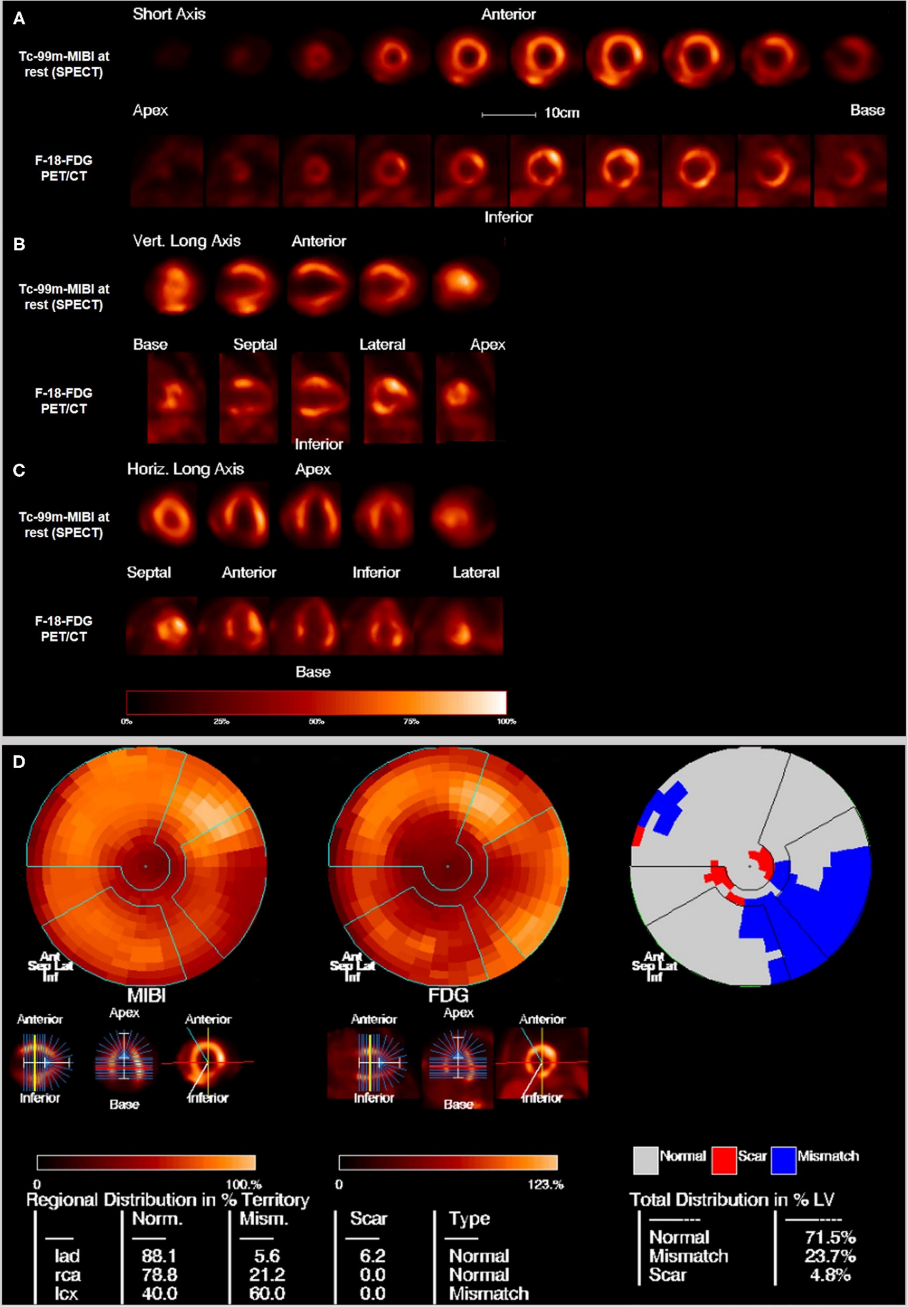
Example of hibernating myocardium: 76 years old male with a perfusion defect on the posterolateral wall in the Tc-99m-MIBI rest scan that shows FDG uptake in the viability F-18-FDG-PET/CT scan resulting in the diagnosis of hibernating myocardium. (A) Short axis, (B) vertical long axis, and (C) horizontal long axis. The upper rows show the perfusion scan at rest with Tc-99m-MIBI (SPECT), the lower rows show the viability scan with F-18-FDG (PET). (D) Polar maps and subtraction image to visualize the mismatch between the perfusion defect on SPECT and the viability on FDG-PET/CT. 23.7% of the myocardium in the left ventricle is in hibernating status.
